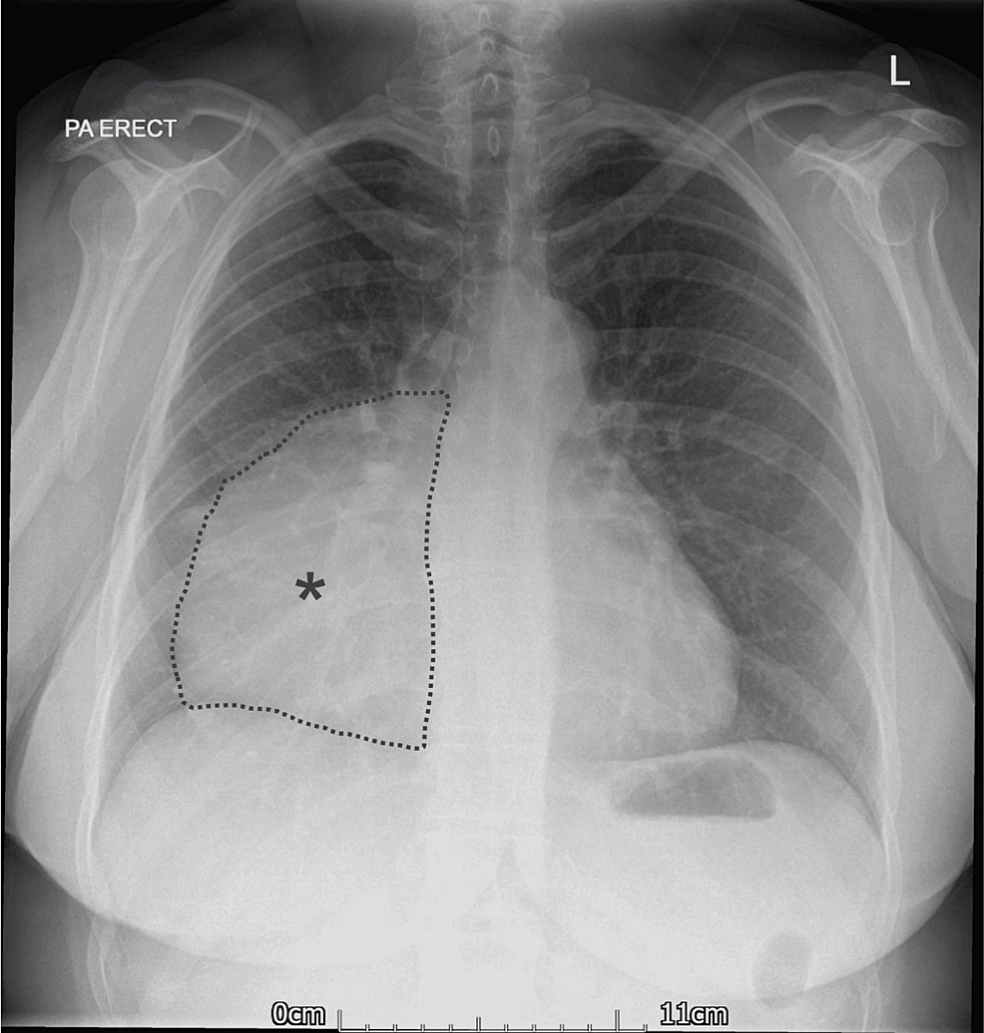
Posteroanterior (PA) chest x-ray showing a soft tissue-density consolidation in the right mid-and-lower hemithorax with obliteration of the right cardiac border (*).
